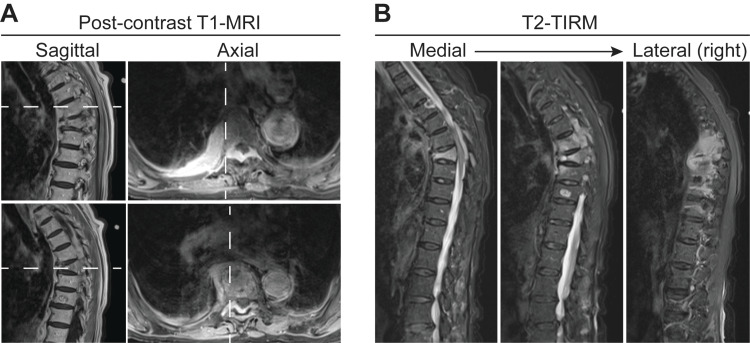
MRI characterization of primary spinal lymphoma with epidural, retropleural, and vertebral body involvement. A) Sagittal and axial post-contrast T1-MRIs demonstrating extensive homogenously enhancing soft tissue mass in the epidural space extending retropleurally through the right neural foramina at multiple levels from T7 – T9. Dashed lines represent relative location of sagittal and axial cuts. B) Fat saturated T2 turbo inversion recovery MRI (TIRM) cuts from medial to right lateral demonstrating hyperintense signal in the T7 – T9 vertebral bodies, indicative of lymphoma extension into the bone. Epidural and retropleural lymphoma appears hypointense to cerebrospinal fluid.
